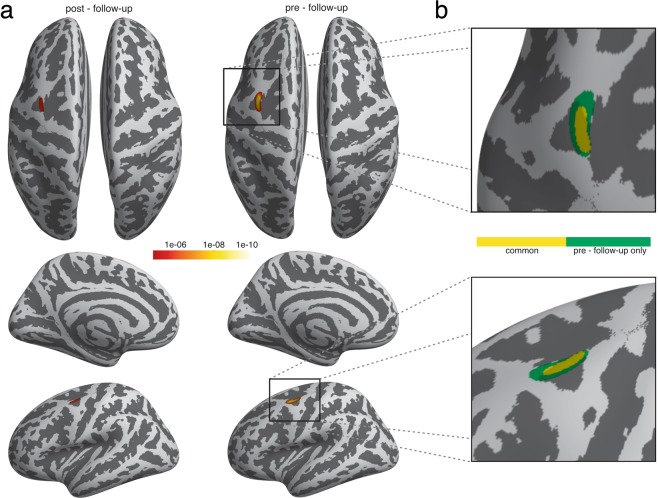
Changes in Cortical Thickness. (a) Increase of CT in the left primary motor cortex. (b) Enlarged view of overlapping increases; yellow (=common): post-test to follow-up, green: pre-test to follow-up.
